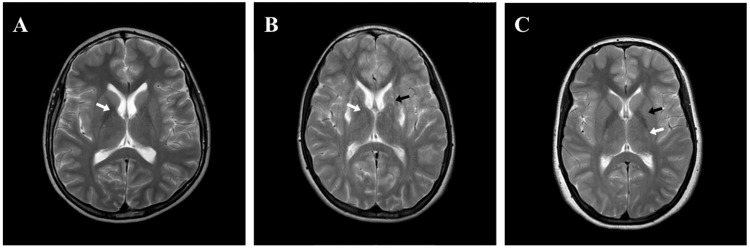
T2-weighted axial brain MRI of patients with Wilson disease. (A) MRI image of Patient 1 showing abnormal hypointense T2 signal intensity at the globus pallidus bilaterally (arrow); (B) MRI image of Patient 2 showing bilateral hyperintensity of the globus pallidus (white arrow) and hypointensity in putamen (black arrow); (C) MRI image of Patient 3 showing high signal intensity at the posterior limb of the internal capsule bilaterally (white arrow) with putamen involvement (black arrow). MRI: magnetic resonance imaging
